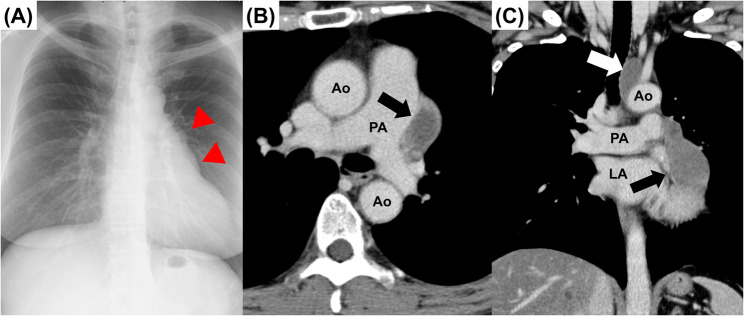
Radiological findings. (A) Abnormal shadow at the left hilum on chest radiograph (red triangle). (B) Horizontal computed tomography image showing a partially encapsulated mass adjacent to the left pulmonary artery (black arrow). (C) Coronal computed tomography image showing a mass at the left hilum (black arrow) and a cyst on the left side of the trachea (white arrow). Ao: aorta; PA: pulmonary artery; LA: left atrium
